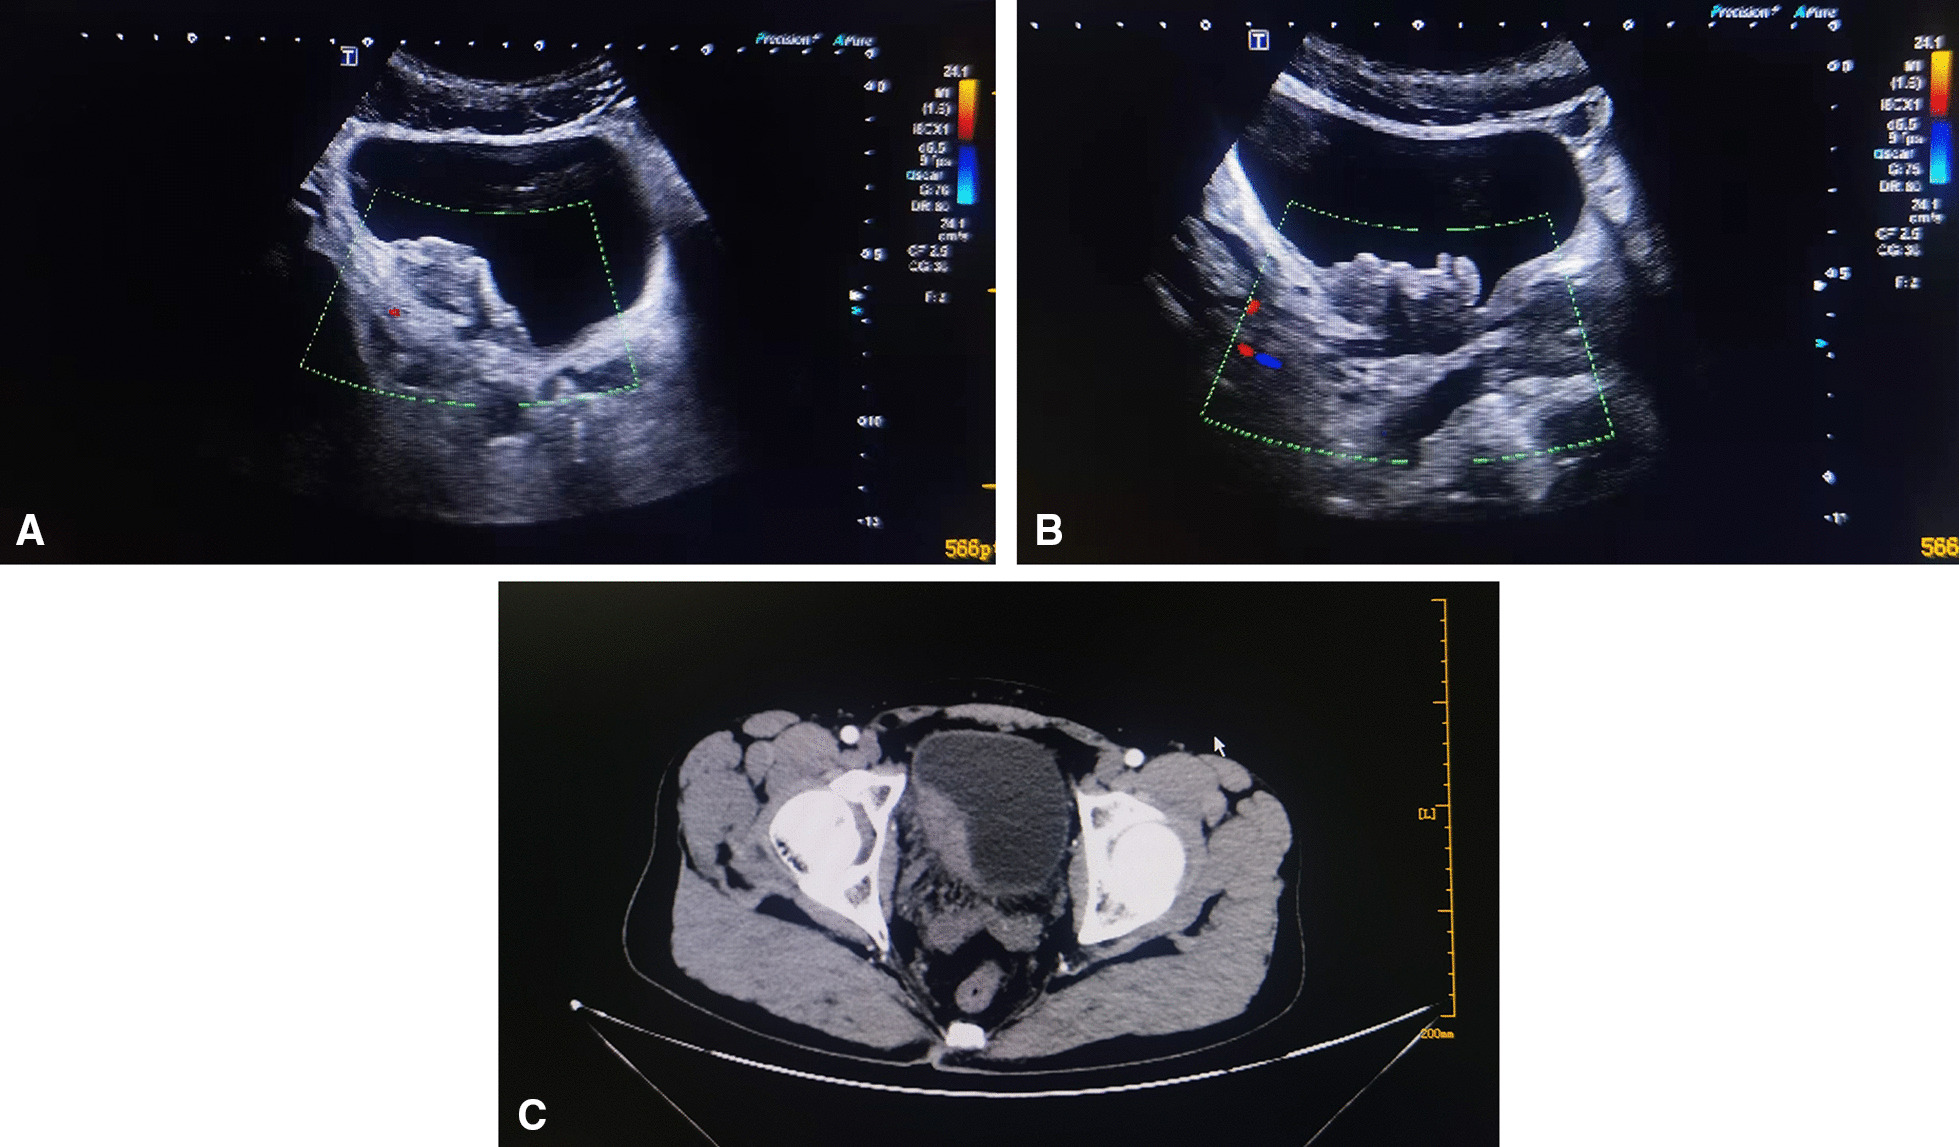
Ultrasound examination. A Low echo on the right portion wall of the bladder. B Low echo on the neck of the bladder; C a remarkable enhancing large mass on the right portion of the bladder with undefined margin
